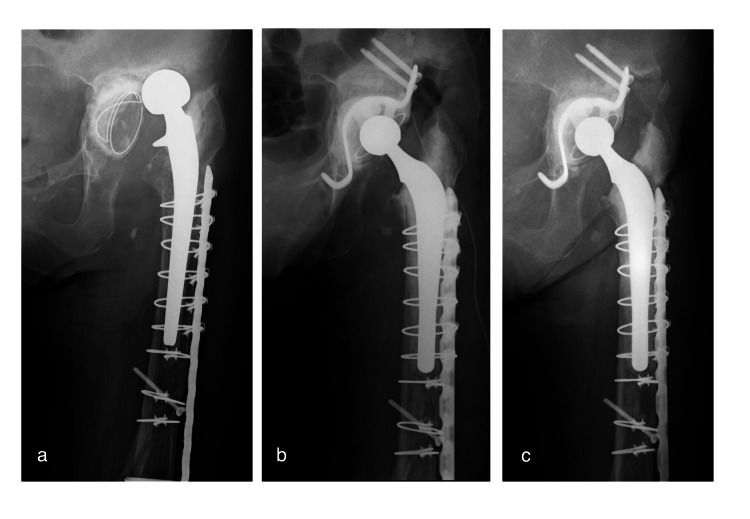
Anteroposterior hip radiographs of an 82-year-old woman who underwent primary THA 14 years ago and revision THA 1 year ago, with rheumatoid arthritis and hemodialysis (case 12, preoperative score of 7 points). (a) Radiograph showing acetabular cup loosening and dislocation due to periprosthetic hip infection; (b) radiograph immediately after one-staged revision THA, including cup and stem; (c) radiograph at four years postoperatively. THA: total hip arthroplasty.
